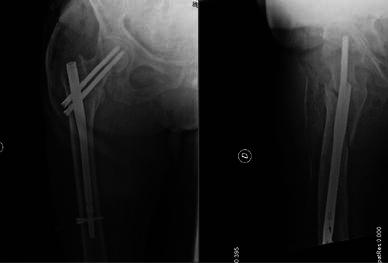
Postoperative radiographs show a medium-sized nail with distal dynamic locking. On the lateral view, the entry site appears to be correct (at the tip of the greater trochanter). Note that the bowing is not that significant
